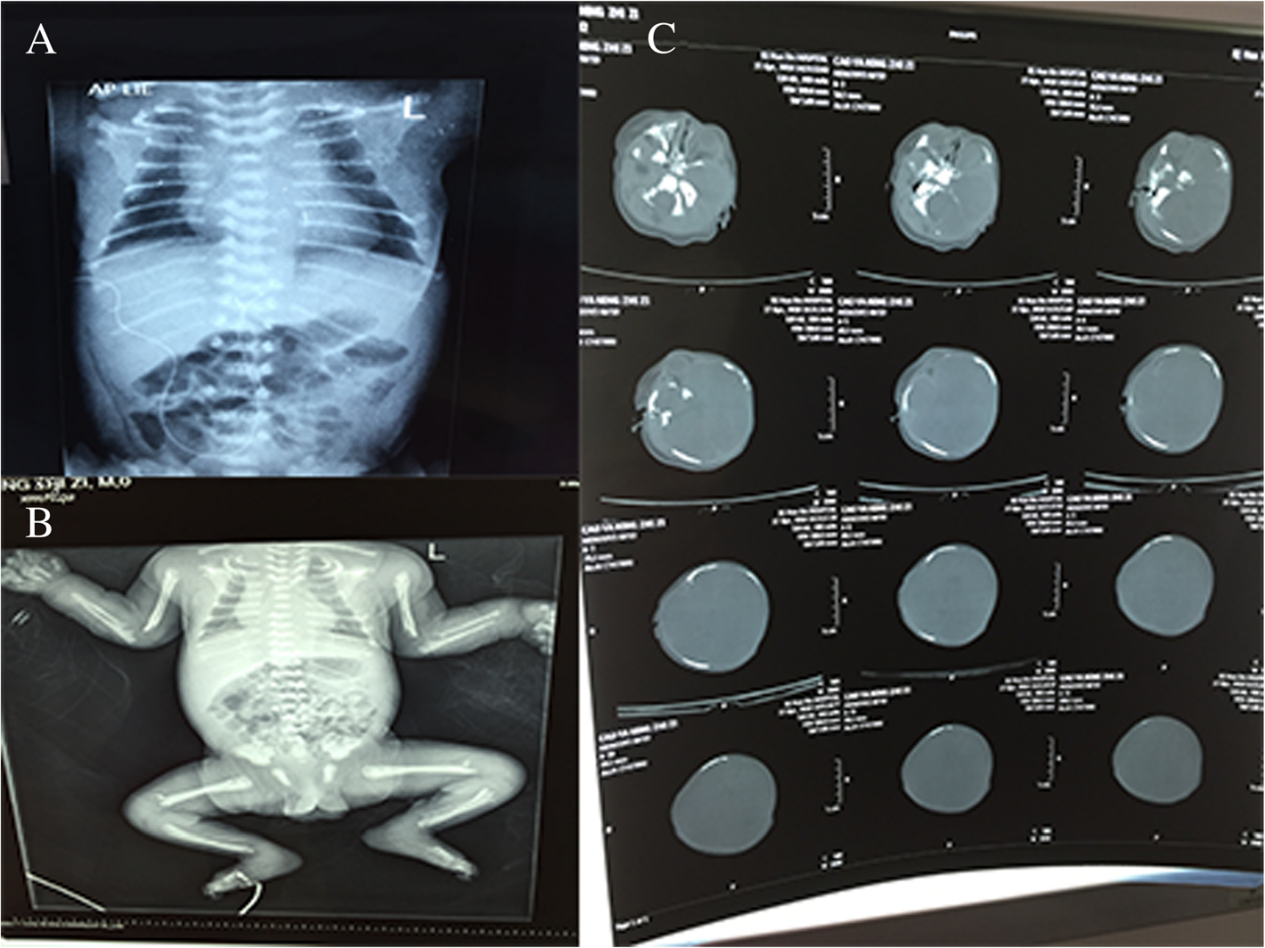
Patient radiography and CT. a thoracolumbar X-ray; (b), long bone of limbs x-ray; (c), patient Head CT
